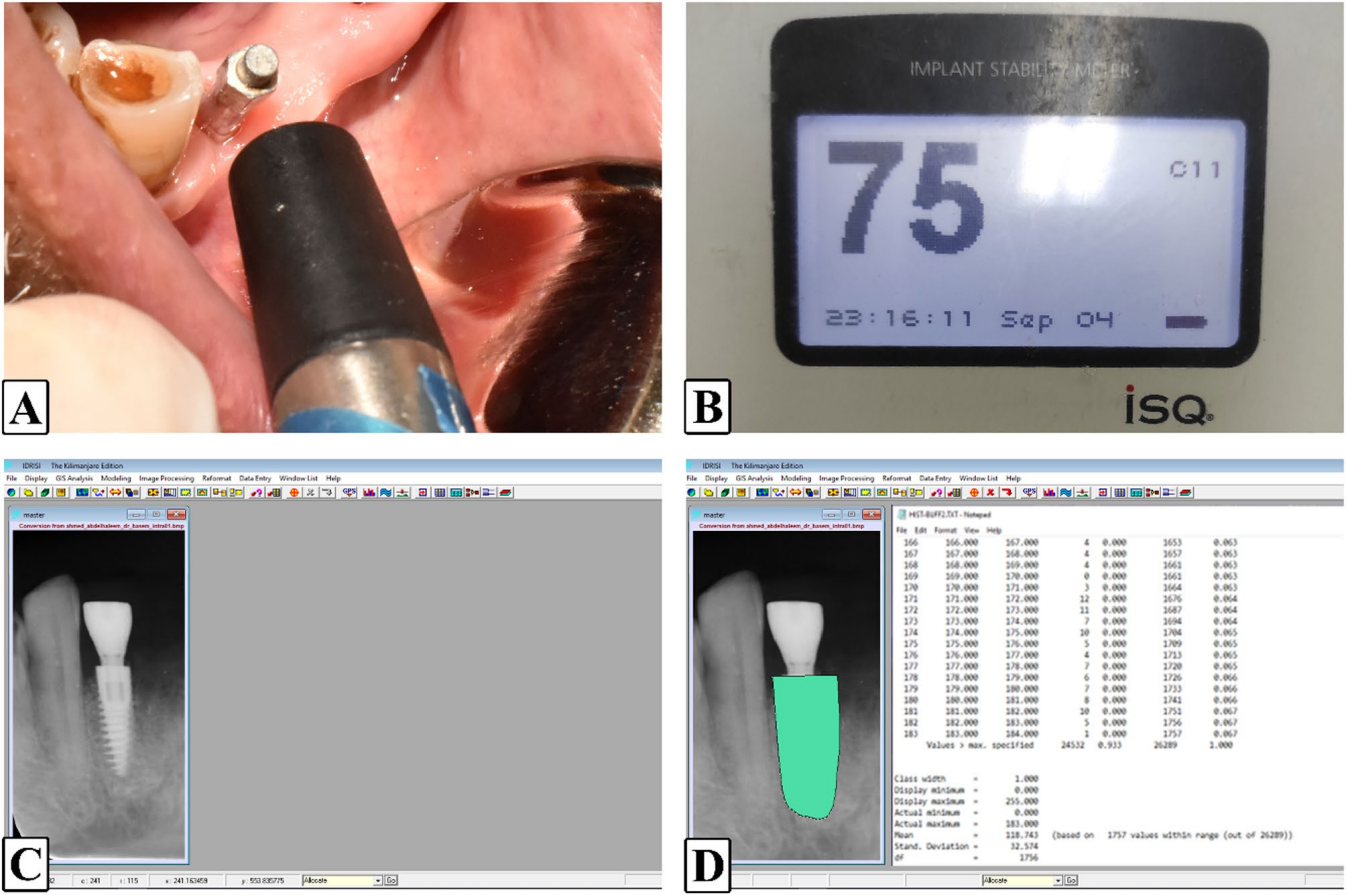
Photographs of post-operative evaluation A. Implant stability assessment with Osstell ISQ device and smart peg B. Osstell ISQ system readings C. Bone density assessment with a digital periapical radiograph using IDRISI Kilimanjaro software D. Bone density values
